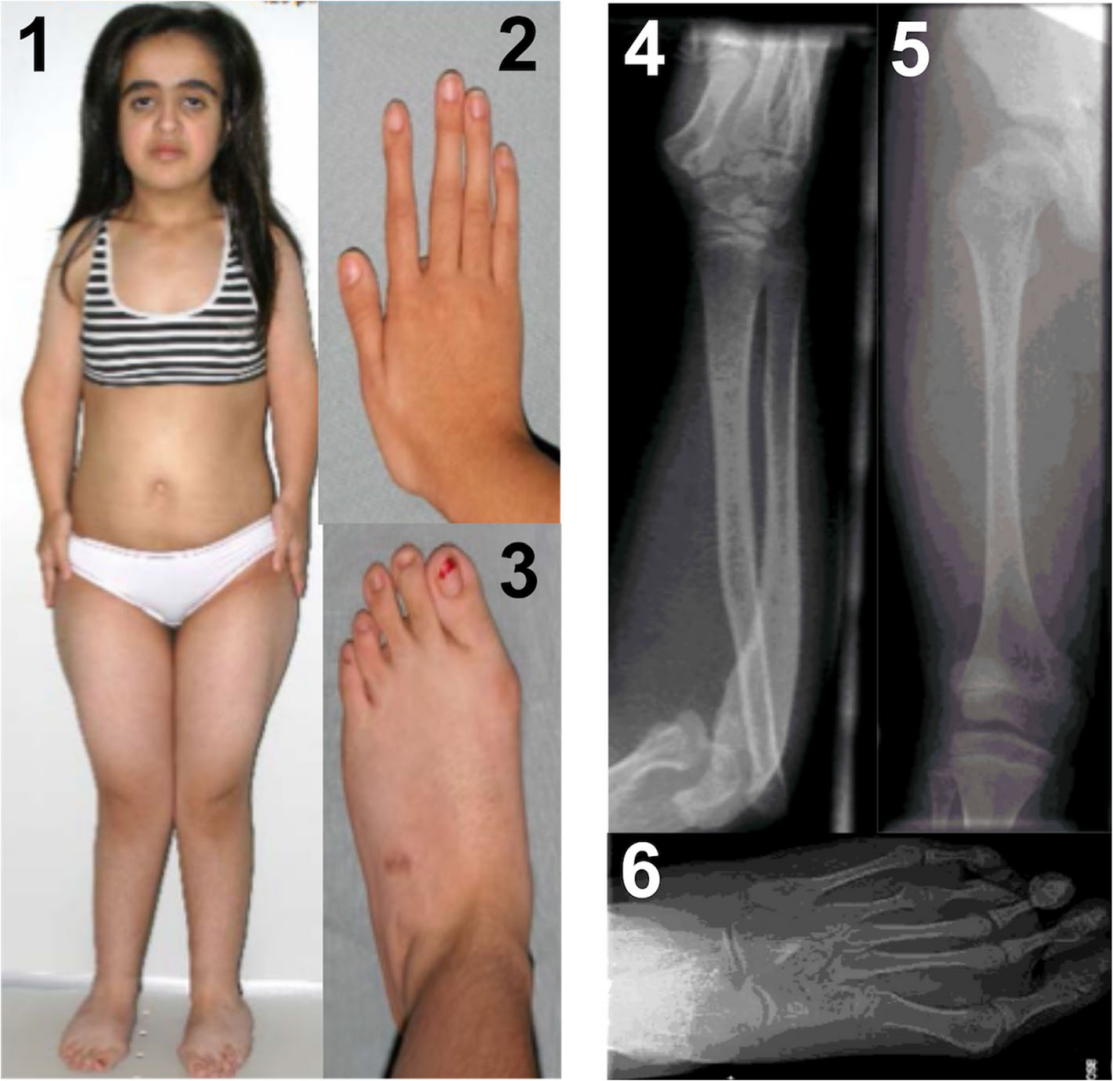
Patient 2 at age 13 yrs. with a disproprionate short stature, genu valgum, a round flat face with midfacial hypoplasia, blue sclerae, downslanting palpebral fissures and prominent eyes [1]. She has long fingers with spatulate distal phalanges and pes planus with hallux valgus [2, 3]. Radiography shows radial head dislocation, short femoral neck, subluxation of the knee joint and irregular tarsal bones [4–6]
